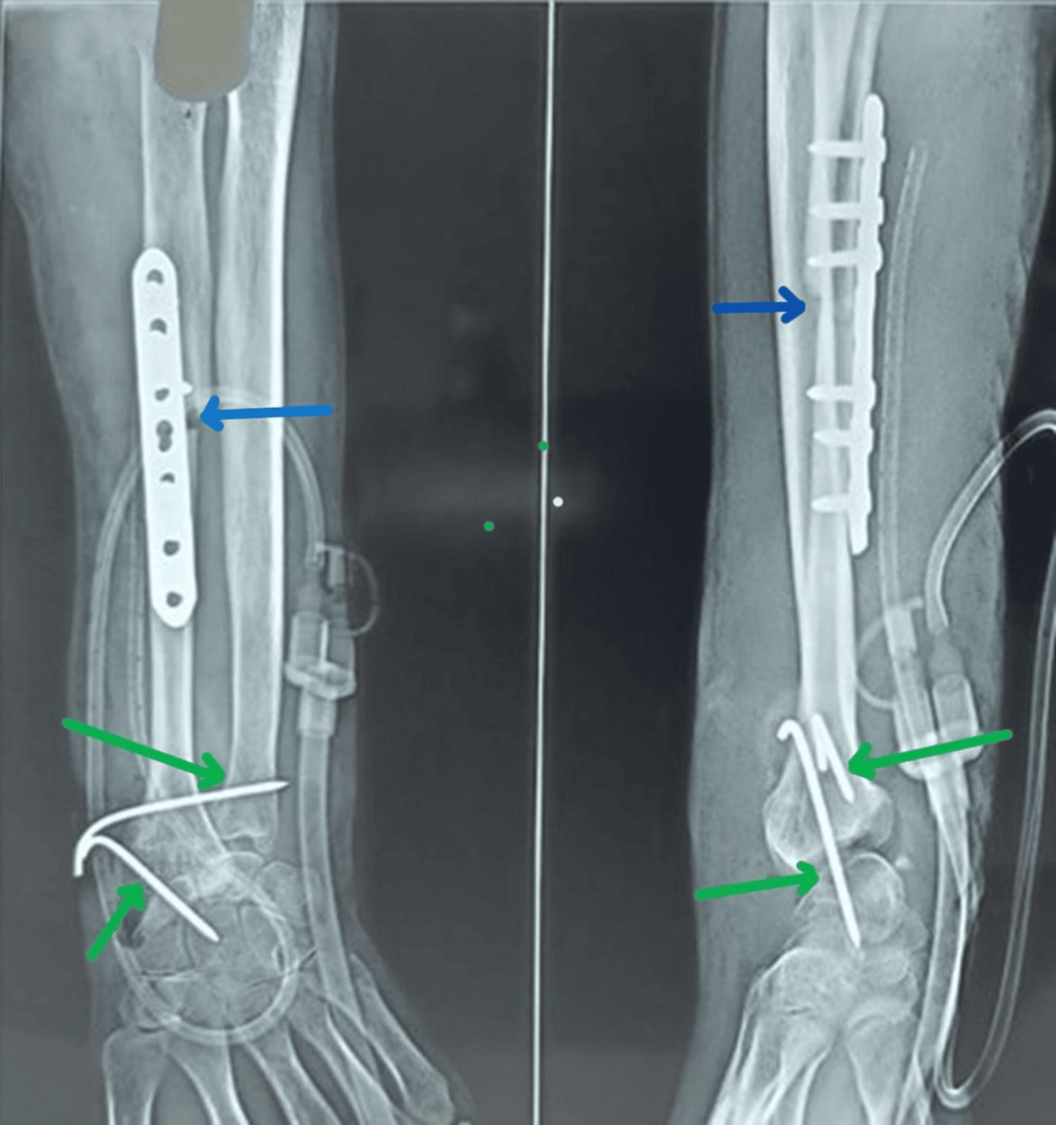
Post-operative radiograph Demonstrates successful tumor resection with fibulo-radial fixation using a 3.5 mm plate (blue arrows) and K-wire stabilization of the fibulo-carpal and fibulo-ulnar joints (green arrows).
